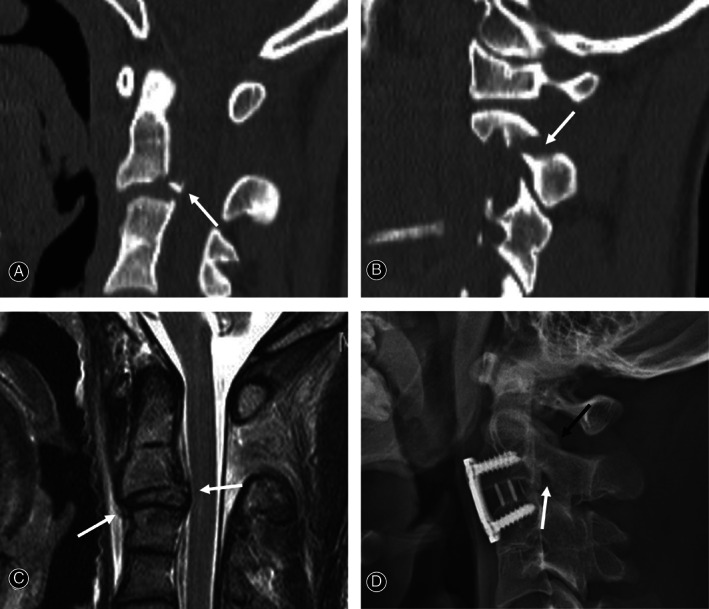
Sagittal and parasagittal computed tomography (CT) scans showing C_2‐3_ anterior slip and posterior tear drop (TD) fracture (white arrow) (A) and hangman's fracture (white arrow) (B). Sagittal magnetic resonance imaging (C) showing C_2‐3_ discoligamentous injuries (white arrows). At 44 months follow‐up after surgery, lateral X‐ray (D) showing solid fusion of C_2‐3_ anterior cervical discectomy and fusion and fractures of pedicle (white arrow) and lamina (dark arrow).
